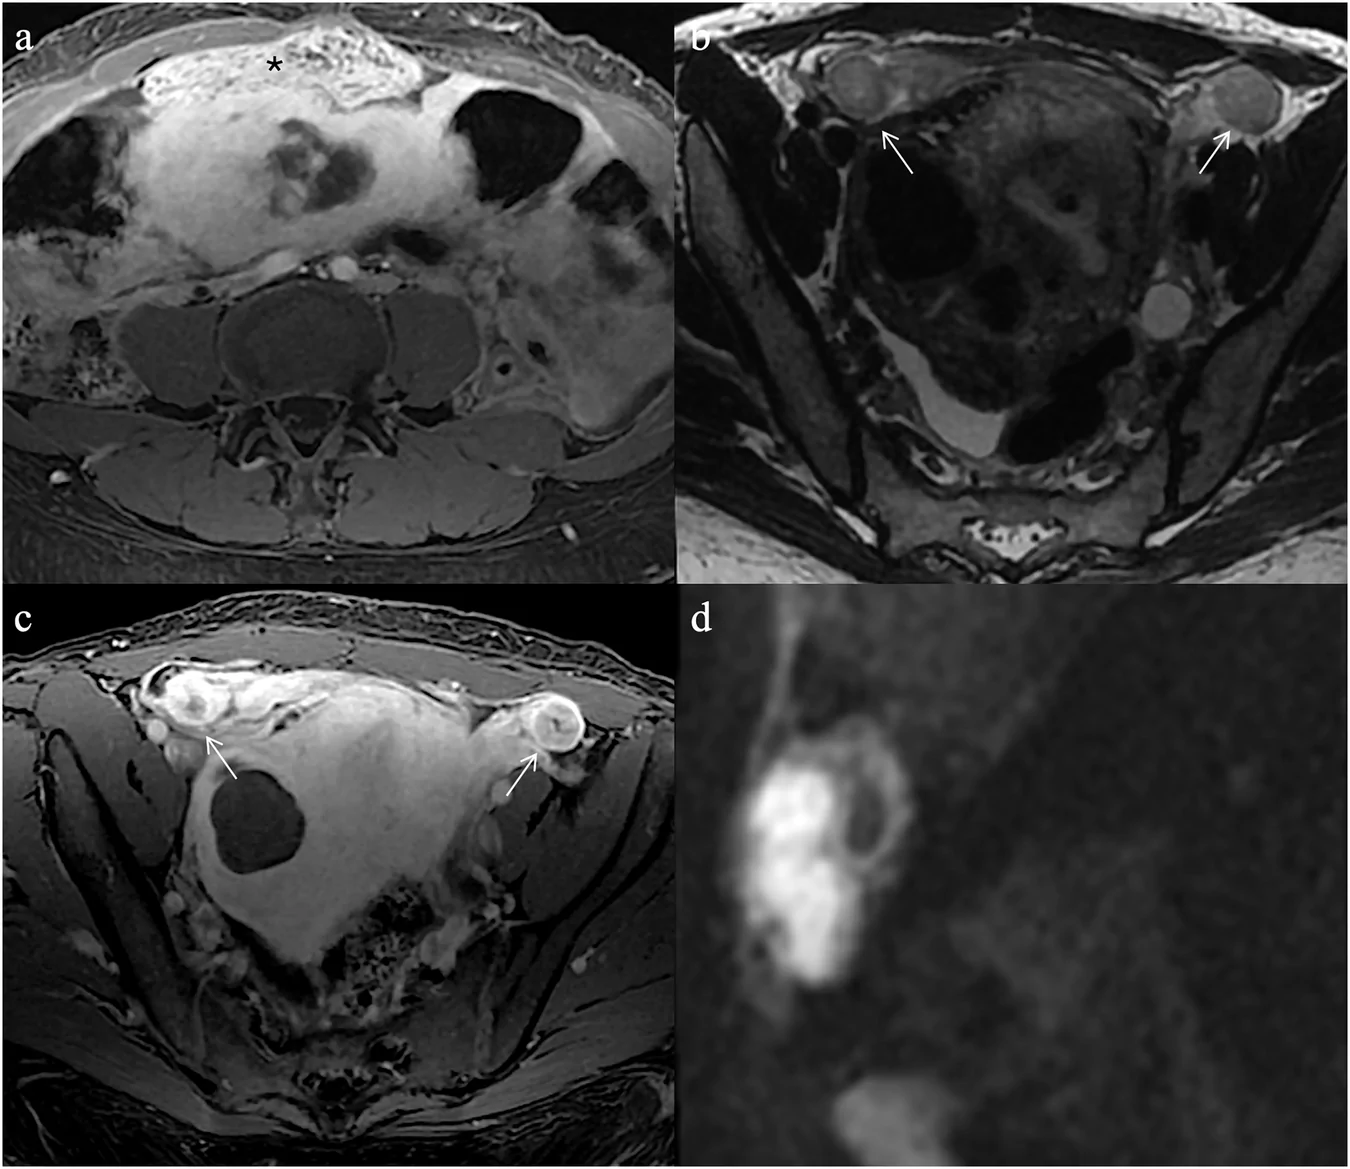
Peritoneal tuberculosis. a Axial postcontrast fat-saturated T1-weighted sequence; b axial T2-weighted sequence; c axial postcontrast fat-saturated T1-weighted sequence; d sagittal DWI sequence centered on the right fallopian tube. A 40-year-old patient was admitted for exploration of peritoneal implants in the context of subacute fatigue with night sweats. The images show omental thickening and enhancement (*), associated with tubal thickening with marked enhancement (white arrows). On diffusion, the right fallopian tube shows marked diffusion signal intensity, suggesting purulent content. Peritoneal tuberculosis was subsequently confirmed
